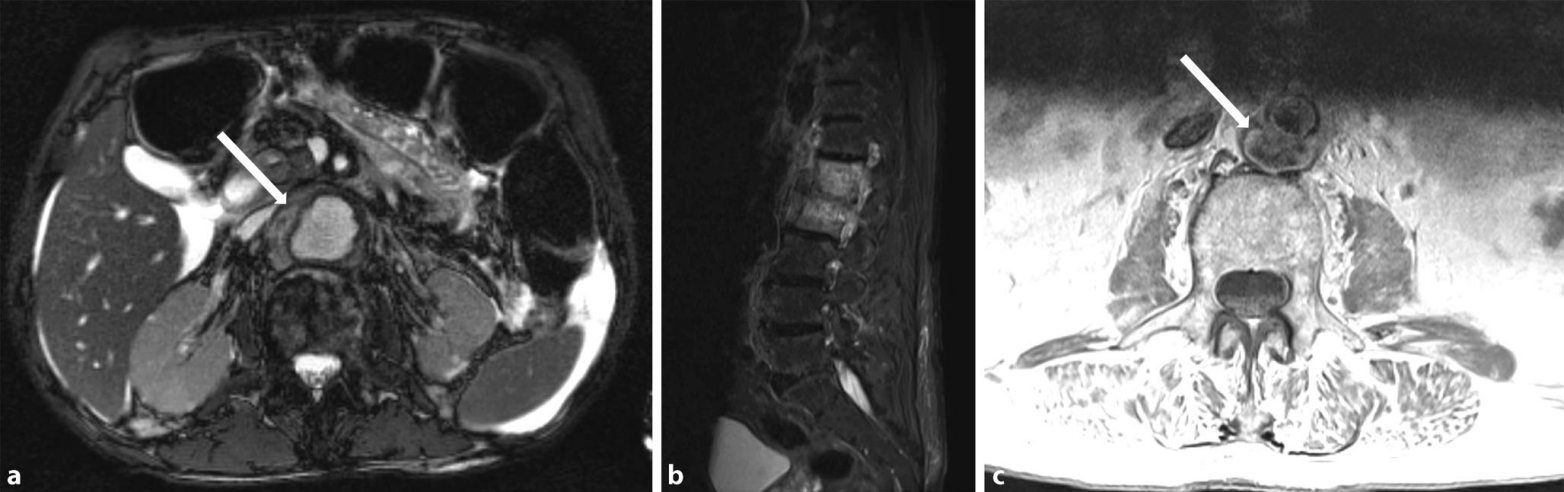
a Magnetic resonance imaging, abdominal (T2-weighted), axial view, marked inflammatory thickening of the abdominal aortic wall (*arrow*), perihepatic and perisplenic free fluid. b Significantly increased signal intensity in lumbar vertebrae 1 and 2 and intervertebral disc consistent with spondylodiscitis and prevertebral cuff of inflammatory soft tissue. c Inflammatory aneurysmal dilatation of the infrarenal dorsal aortic wall (*arrow*) in direct connection with the vertebral body
